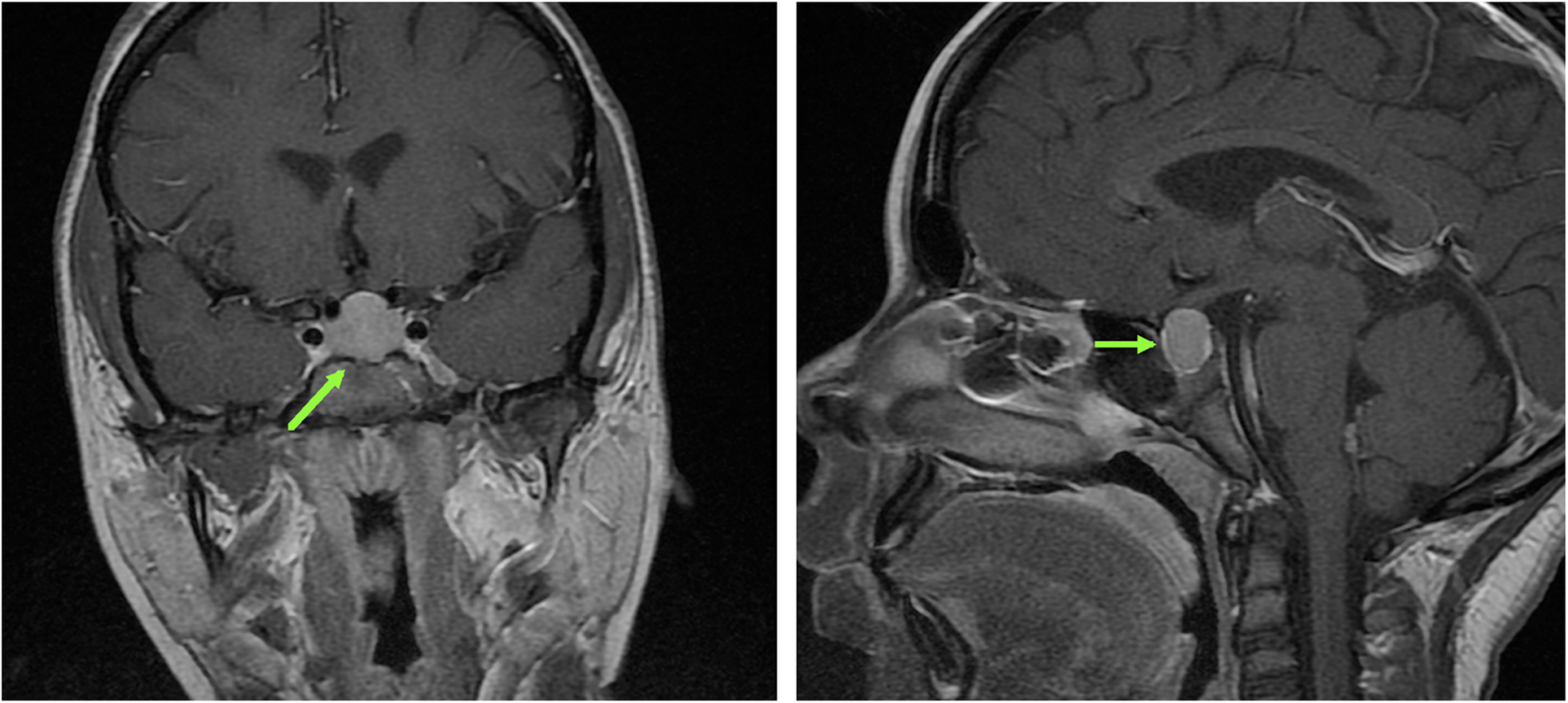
Enlarged pituitary gland at presentation. The enlarged pituitary gland measured 1.4cm in size and extended into the suprasellar cistern (green arrows, coronal and sagittal views on T1-weighted post-gadolinium images).
